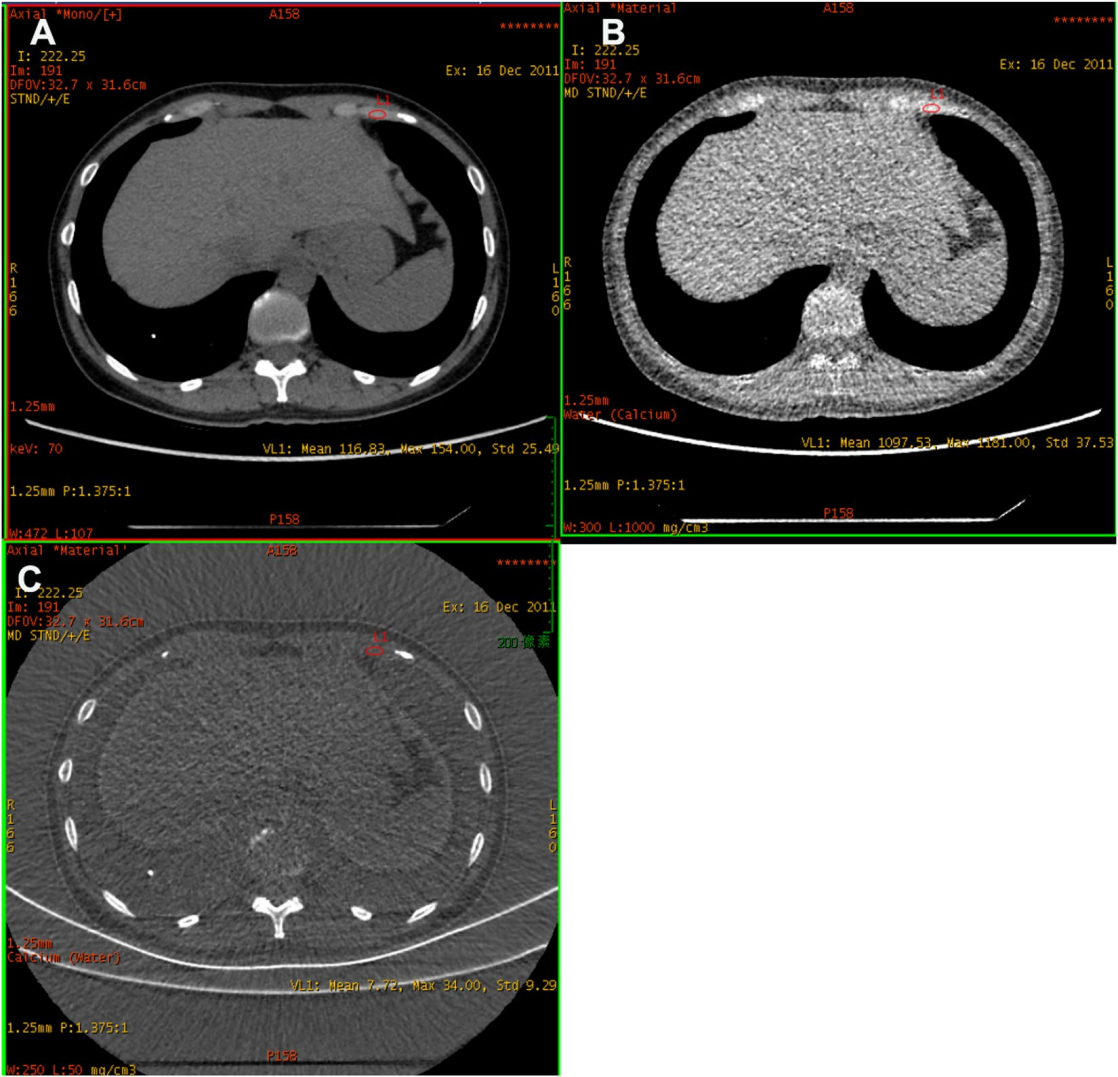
The evaluation of costal cartilage using DECT. (A) The CT value of costal cartilage. (B) The water concentration of costal cartilage. (C) The calcium concentration of costal cartilage.
